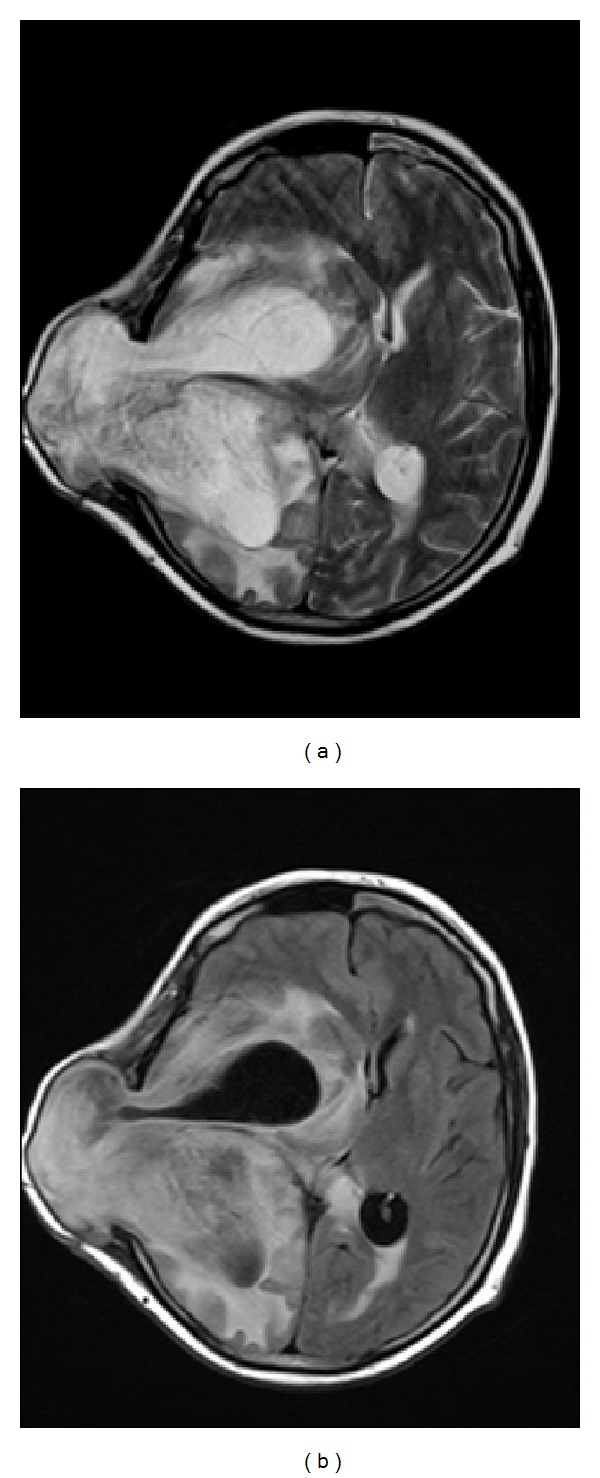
T2 weighted (a) and (b) FLAIR MR images of the lesion before the delivery on axial plane showing a recurrent lesion at the same location. In this figure we see herniated parenchymal tissue from craniectomy defect. There is midline shift about 18 mm in diameter. Mesencephalon and pons retracted to left and there is also uncal herniation.
